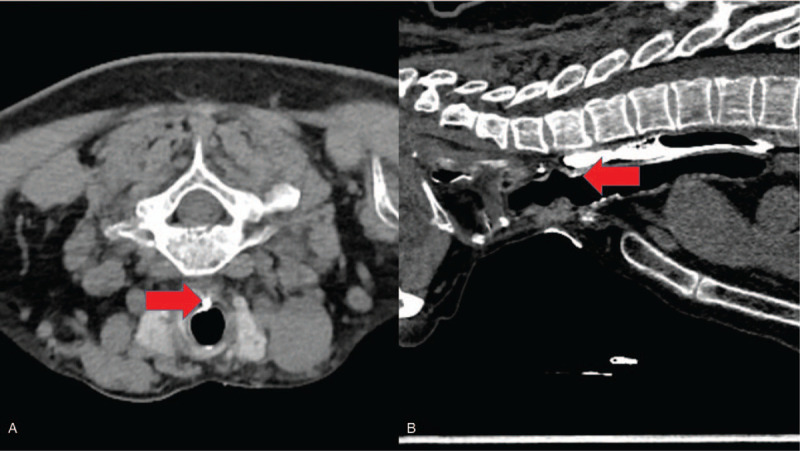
Computed tomography chest images in prone position presenting contrast leakage at the tracheoesophageal fistula (TEF) (red arrow) (A) Axial view (B) Sagittal view.
